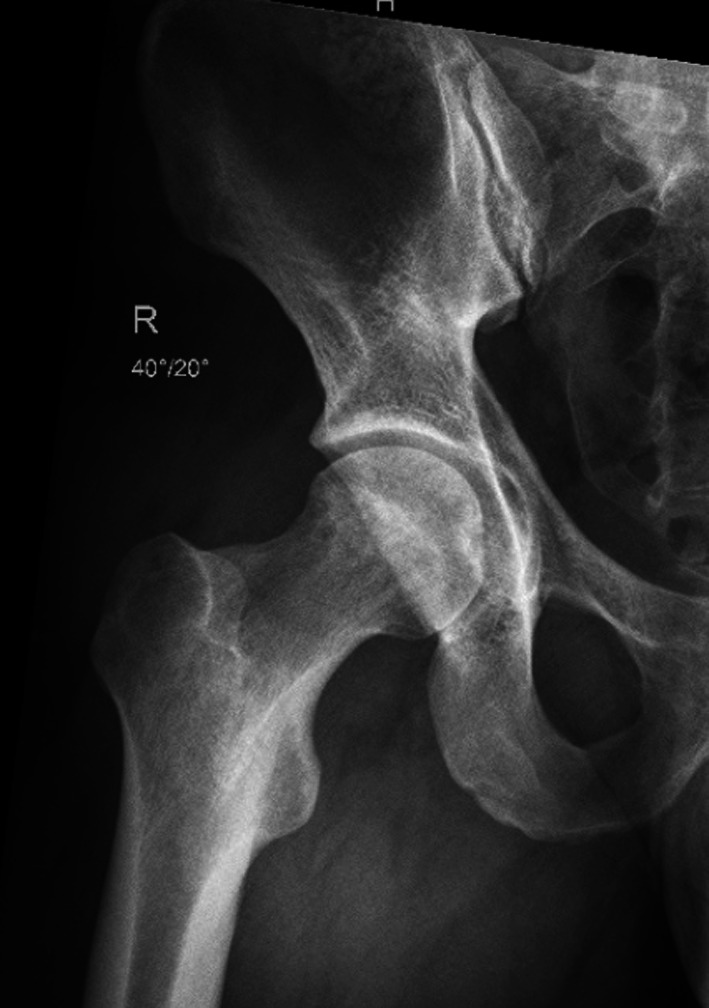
Routine postoperative radiograph with a modified Dunn view following right-sided femoral osteoplasty for the same patient
